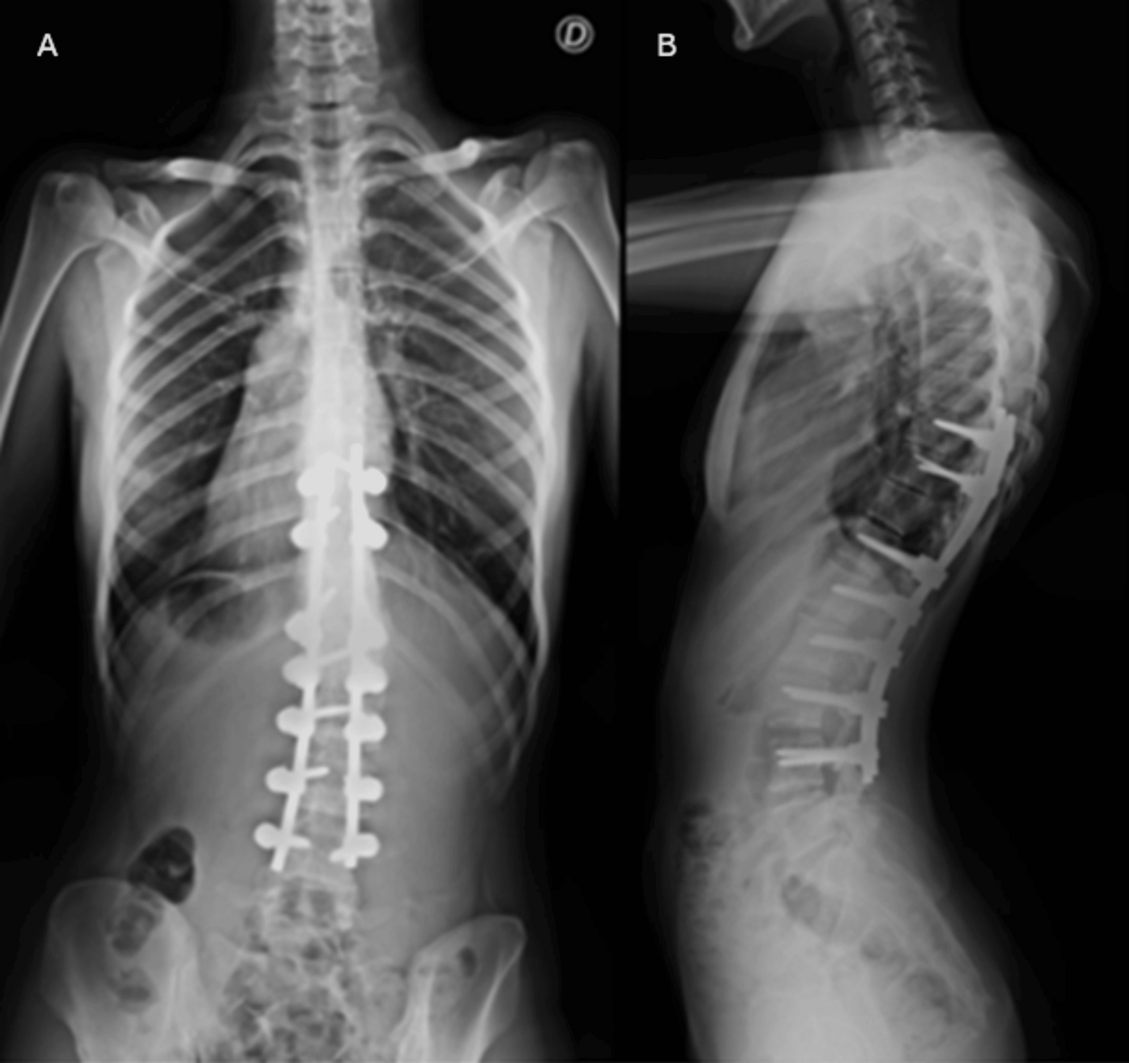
(A) Axial and (B) lateral X-ray of the patient's spine Both figures highlight surgical material following posterior spinal surgery from T9 to L4. In the figures, we can see that several vertebral bodies have fixation screws and that two longitudinal rods extend from T9 to L4. The presence of this surgical material may hinder the search for the epidural space.
